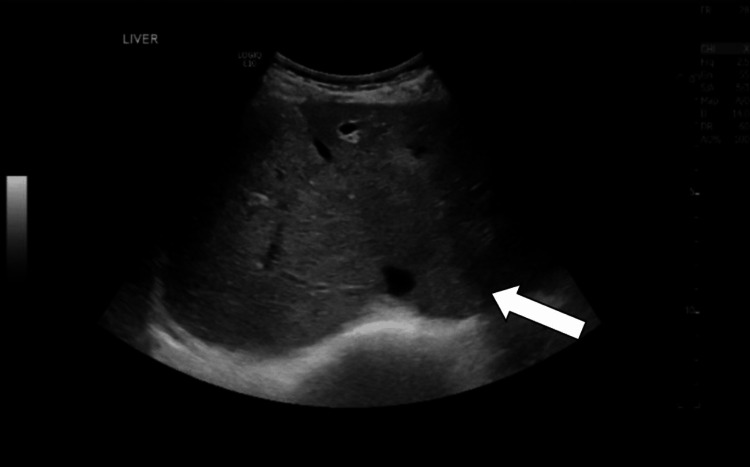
Ultrasound image obtained before colonoscopy showing the liver (arrow) with normal appearance.
